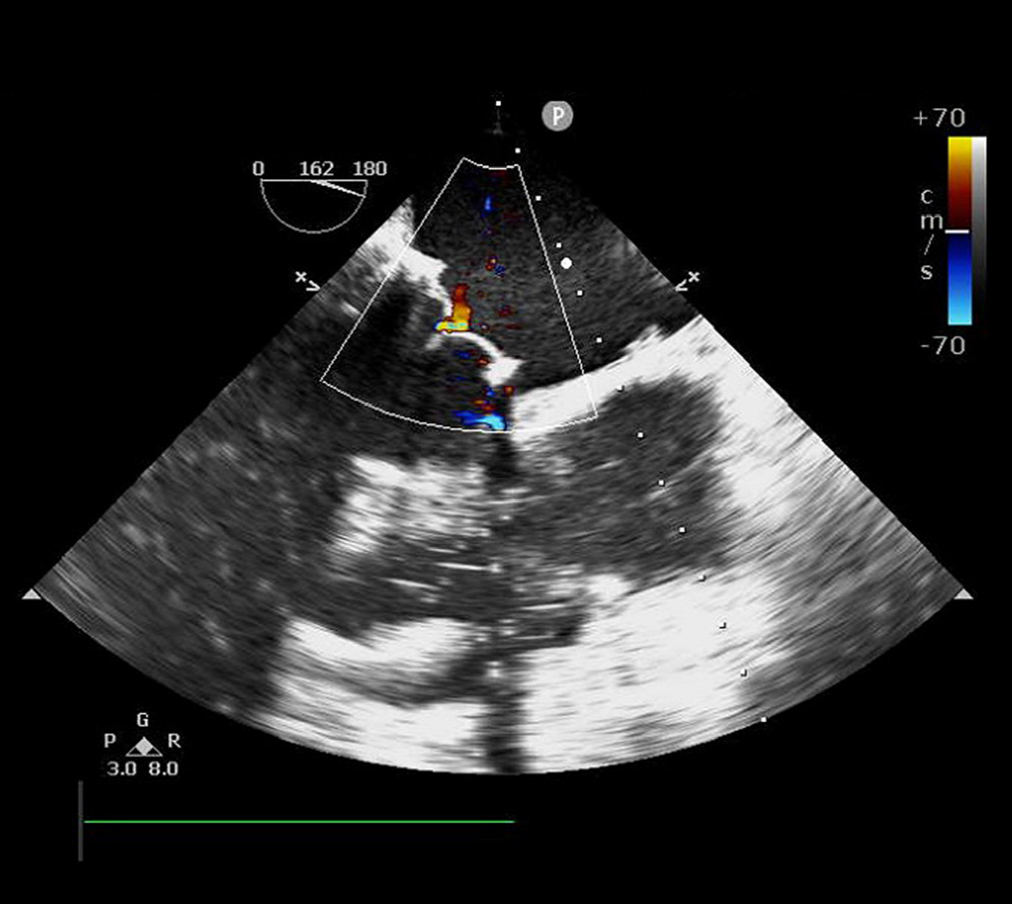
Intraoperative transesophageal echocardiography showing no leakage.
